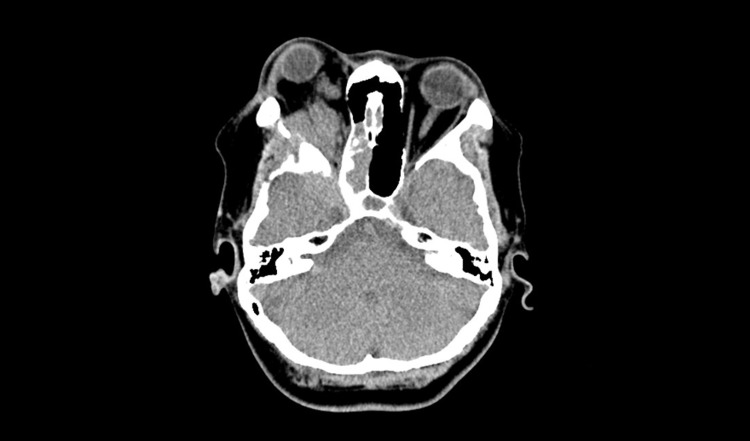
Axial post contrast computed tomography image demonstrates sphenoid wing meningioma with extension into right optic canal, right posterior ethmoid and sphenoid sinuses posteriorly. Captured seven months prior to third surgery.
